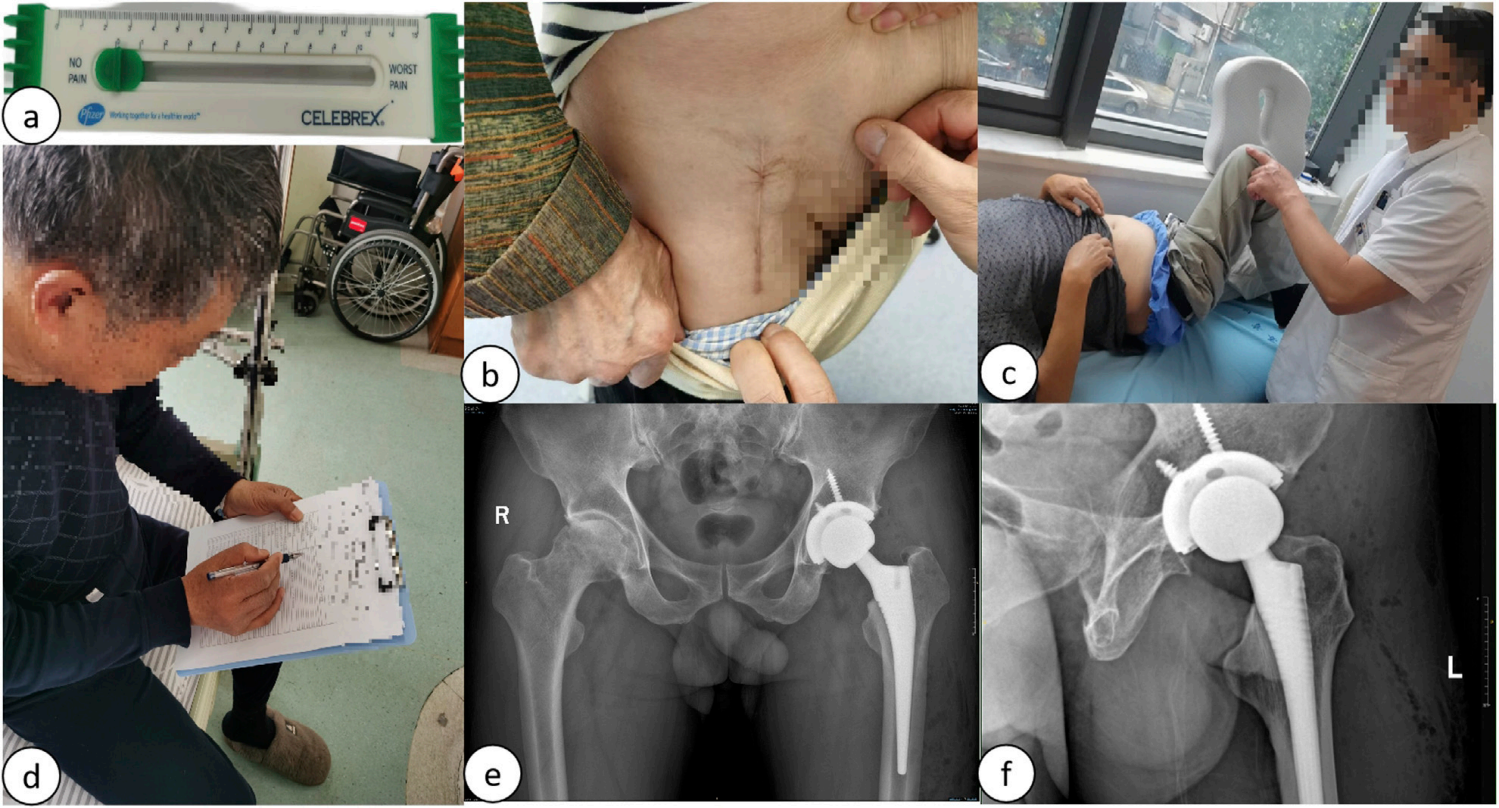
(A) VAS score measurement; (B) Follow-up of incision scars 6 months postoperatively; (C) Follow-up of functional range of motion 6 months postoperatively; (D) Follow-up of postoperative Harris Hip and WOMAC scores; (E) Follow-up of postoperative bilateral hip joint anteroposterior radiographs; (F) Follow-up of postoperative hip joint lateral radiographs.
